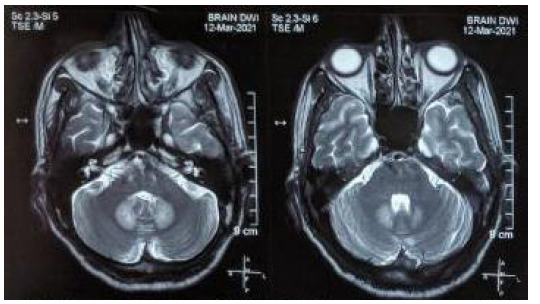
MRI of the brain showing symmetrical high signal intensity in the dentate nucleus of the bilateral cerebellum.
